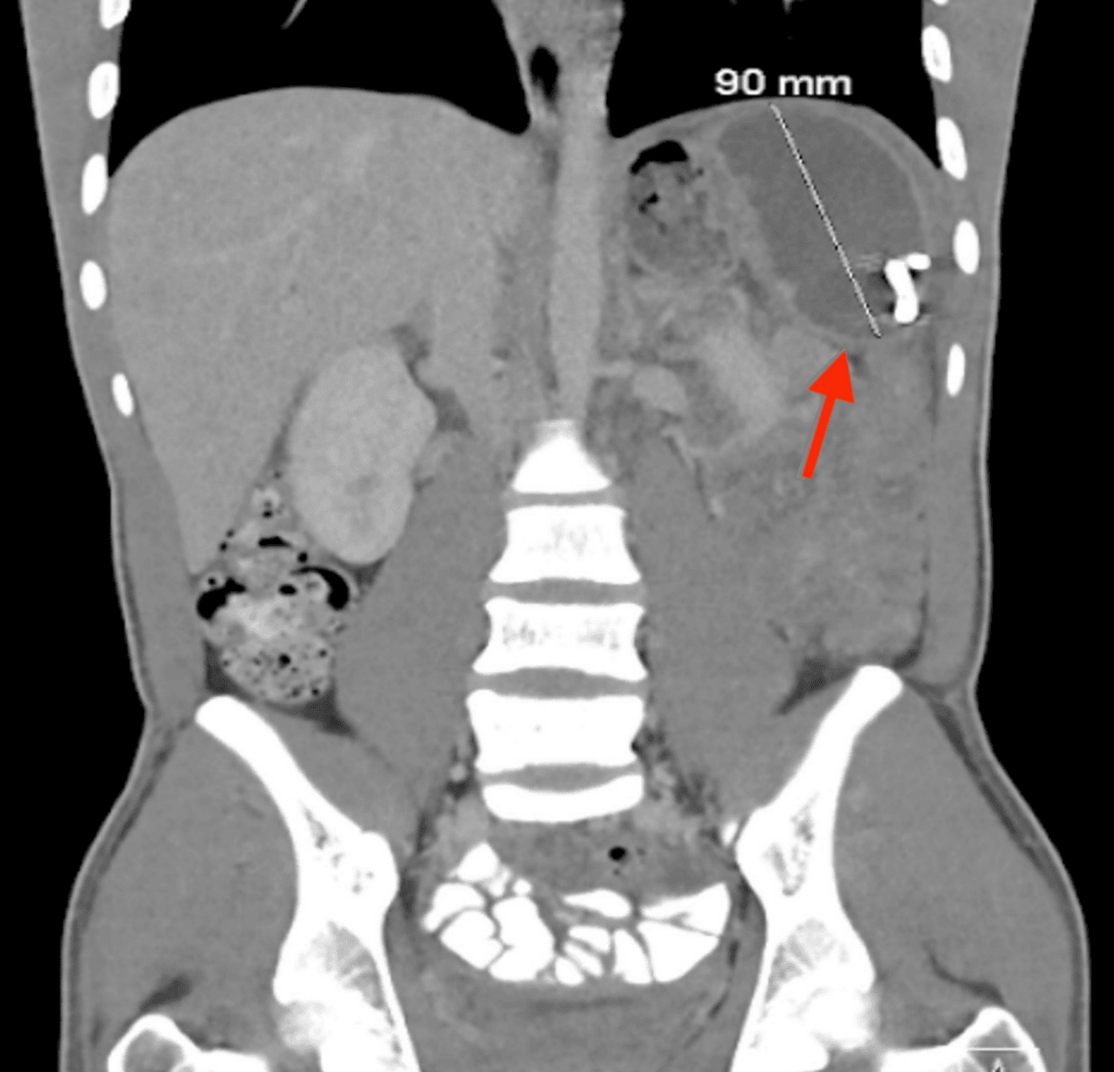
Coronal view CT abdomen/pelvis after percutaneous drainage Coronal view CT abdomen/pelvis showing a rim-enhancing heterogeneous lesion within the spleen, now measuring approximately 7.5 x 5.0 x 10.0 cm after percutaneous drainage.
